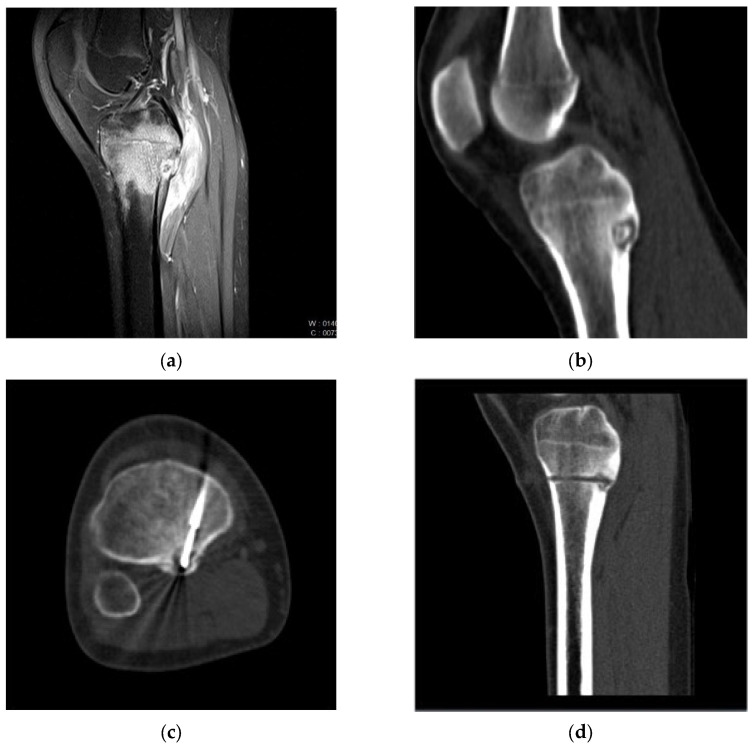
Pre-treatment CT (a) and MRI (b) images of a cortical Osteoid Osteoma in the posterior aspect of the tibia in a 14-year-old girl. (c) CT image during cryoablation: anterior access was performed to reach the “nidus” with the cryoprobe, aiming to avoid the neurovascular bundle. (d) Post-procedure CT image showing the trace of the Bonopty introducer needle path within which the cryoprobe is positioned.
